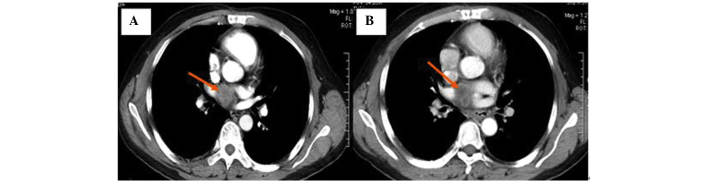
(A) Representative CT image (3/20/2013) showing recurrence of the pulmonary artery leiomyosarcoma tumor (arrow). (B) Representative CT image during radiotherapy (04/16/2013) showing a slight reduction in the size of the tumor (arrow). CT, computed tomography.
